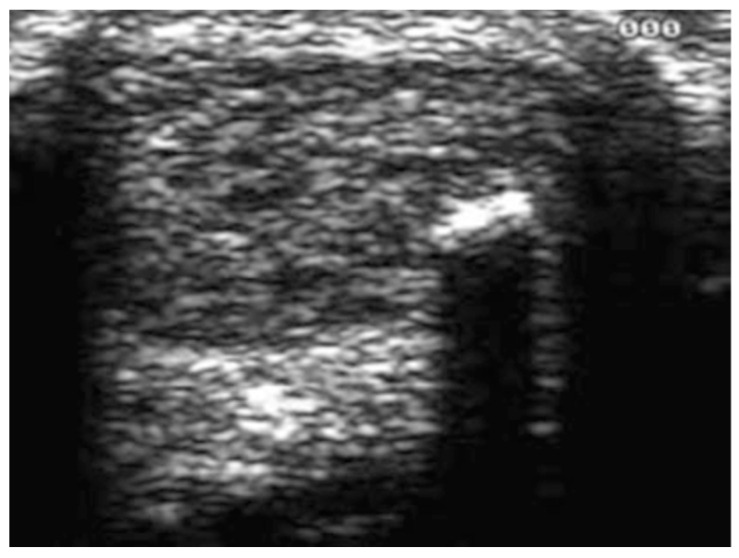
Ultrasonographic appearance of Achilles tendinopathy in a 28-year-old male soccer player at presentation. The longitudinal scan shows that the tendinopathic tendon is thicker than the asymptomatic contralateral one. The normal, well-ordered fibril distribution is lost.
